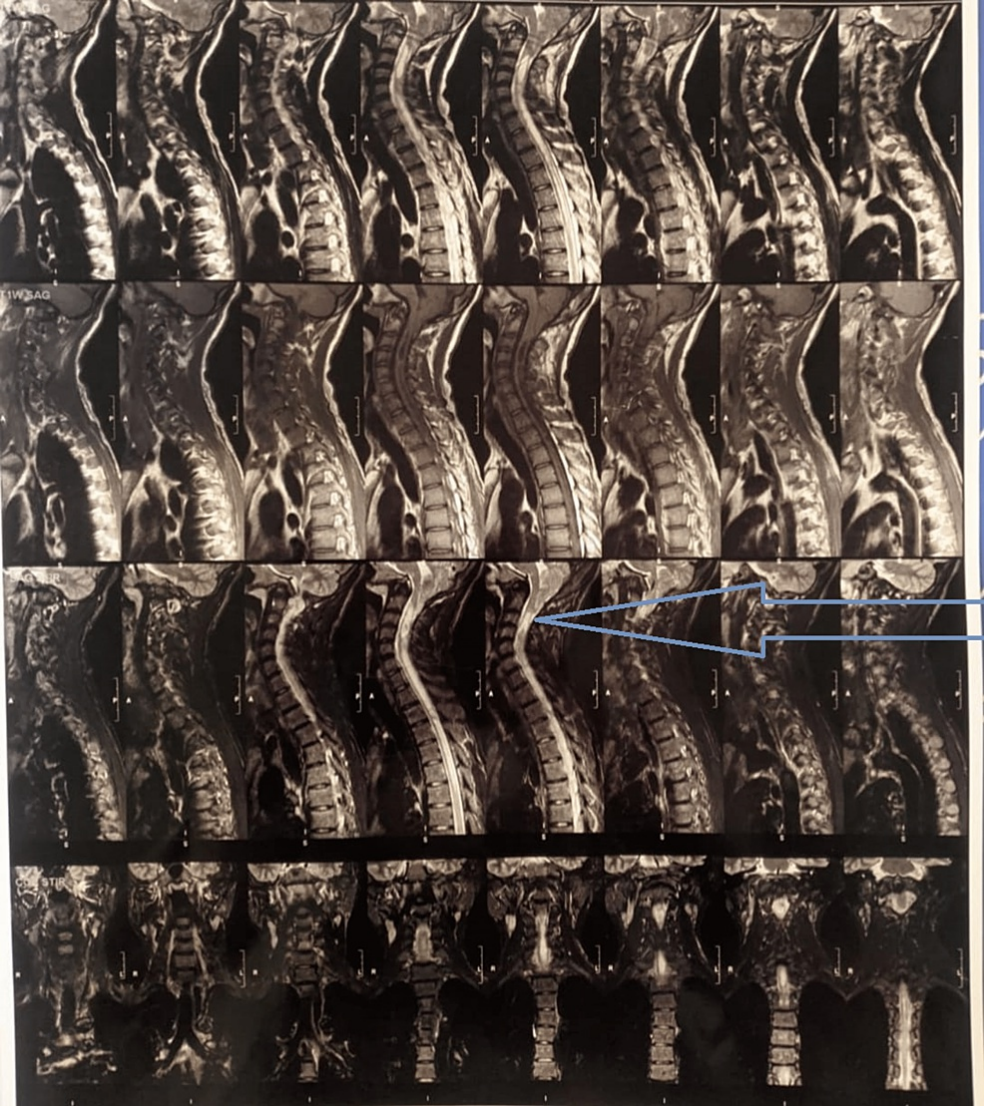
MRI of the spine showing evidence of displacement of the medulla, fourth ventricle, and cerebellar vermis through the foramen magnum.
